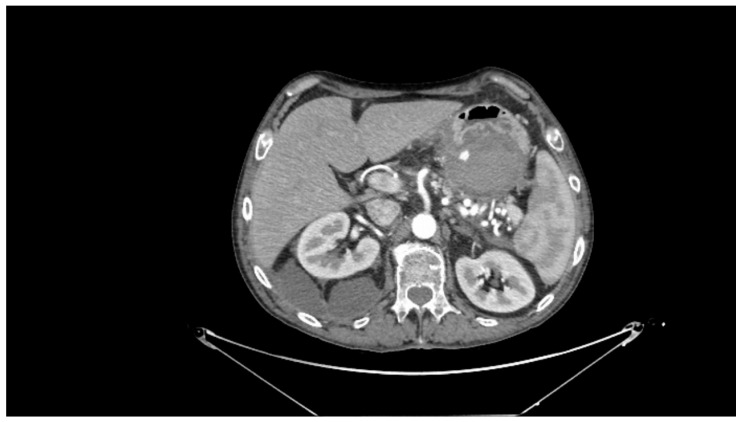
Contrast-enhanced abdominal computed tomography showing active bleeding inside a peripancreatic collection. Reproduced with permission from Bellio, G., et al. [29].
